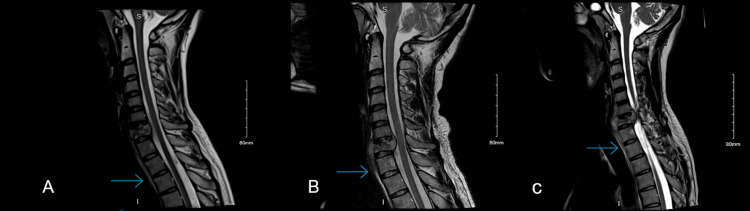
(A) Initial MRI imaging showing C7 destruction and collection extending to upper border of T3. (B) This is the second MRI brought by the patient at follow-up less than 3 weeks after the first one, in which despite ongoing destruction we can see regression in the size of the collection to the inferior border of T1. (C) MRI done pre-op which was done about 2 weeks after, which showed further destruction and stenosis of the spinal canal, however the collection size remained almost similar to the follow up MRI.
